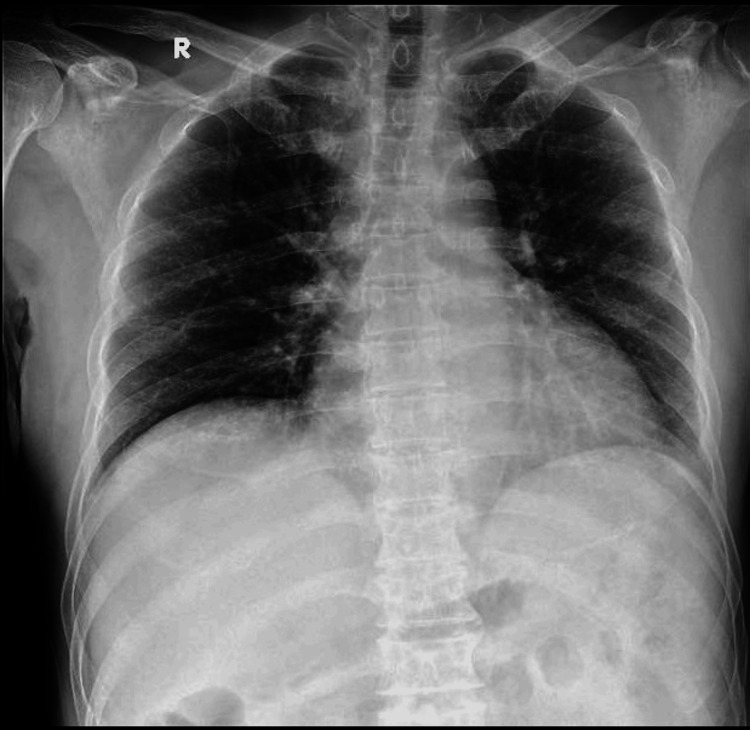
Chest radiograph showing an elevated right hemidiaphragm.
